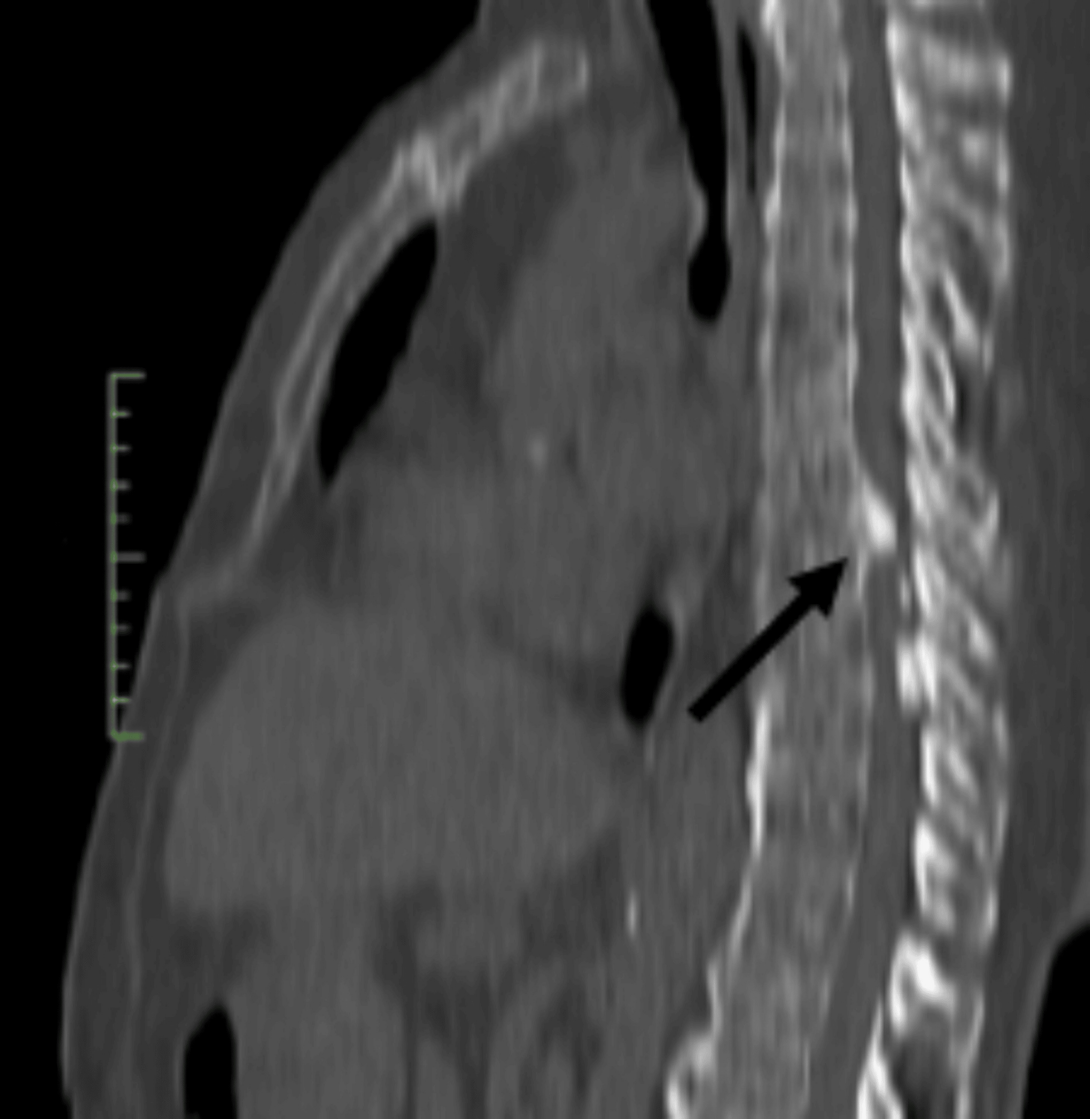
CT scan of the thoracic spine showing evidence of calcification of an extruded herniation at the T7-T8 level in a sagittal view (black arrow).
